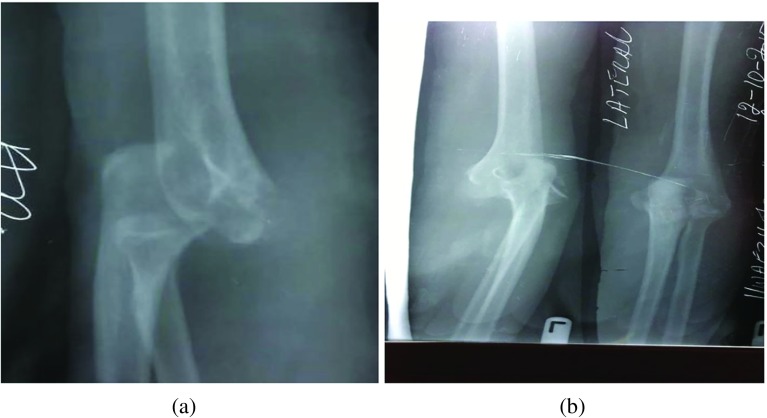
(a and b) The anterior–posterior and lateral X-rays of a patient showing unreduced elbow dislocation. Note the disruption of the radio-capitellar line in the lateral view.
